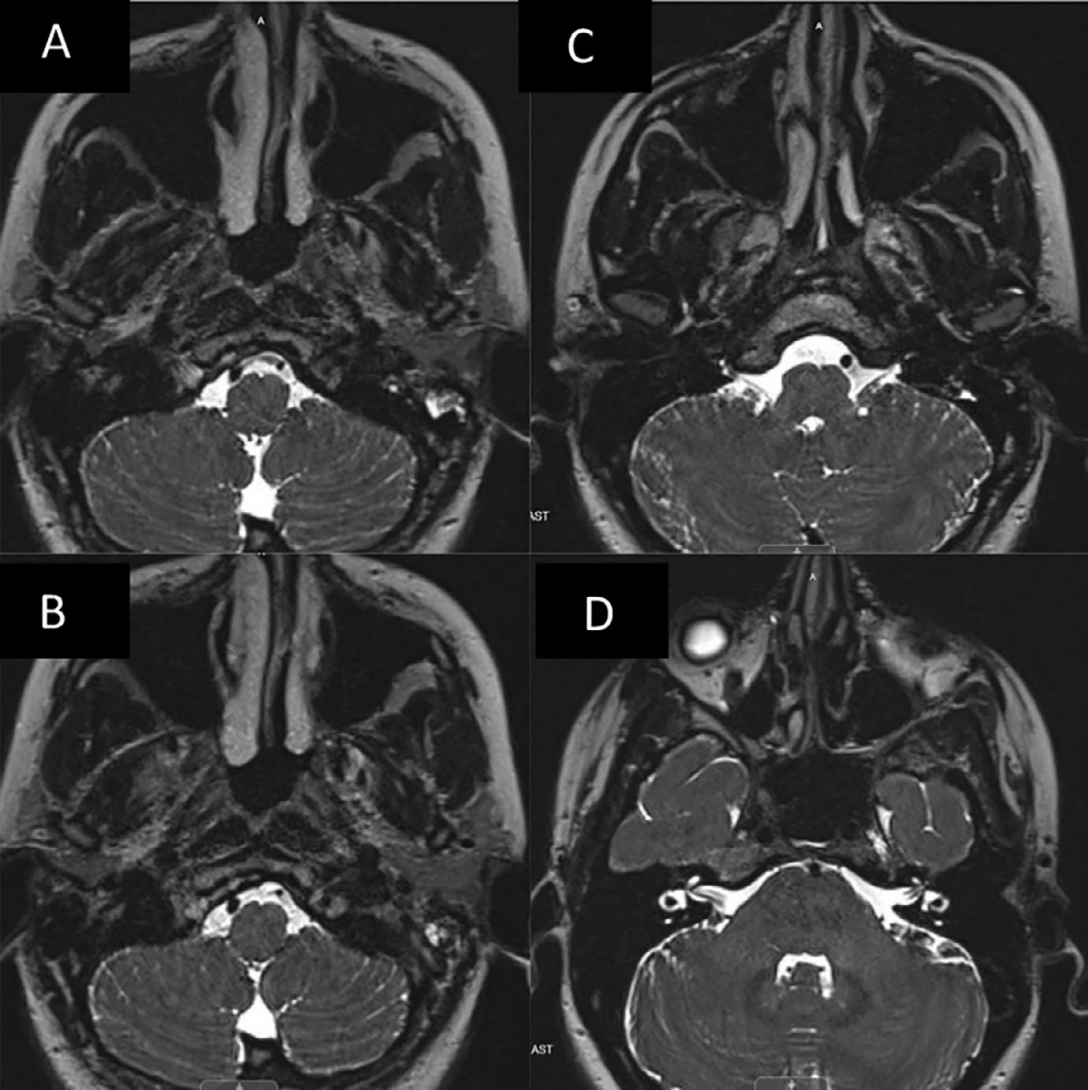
A‐D MRI brain w/o contrast, T2 weighted imaging showing (A and B, left images) increased edema of the right hypoglossal nerve compared to left as it enters the hypoglossal canal, and (C and D, right top/bottom images) cranial nerves 9–11 exiting the jugular foramen and 7–8 exiting the IAC for comparison.
